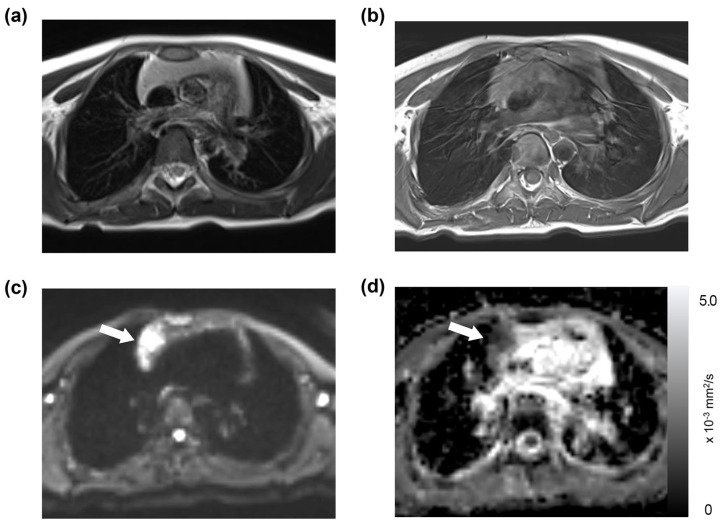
Focal restricted diffusion in the thymus: a 5-year-old girl with a history of mediastinal ganglioneuroblastoma. This patient underwent an MRI on a Siemens B scanner, with their laboratory data showing a WBC count of 8.13 × 10^3^/μL, an absolute neutrophil count of 2.89 × 10^3^/μL, an absolute lymphocyte count of 3.94 × 10^3^/μL, and a platelet count of 216 × 10^3^/μL. The thymus was found to be slightly hyperintense on the T2-weighted image (a) and isointense on the T1-weighted image (b). Focal restricted diffusion was present on the DWI (c, arrow), correlating with low-signal intensity in the ADC map with a mean ADC value of 1.04 × 10^−3^ mm^2^/s (d, arrow).
